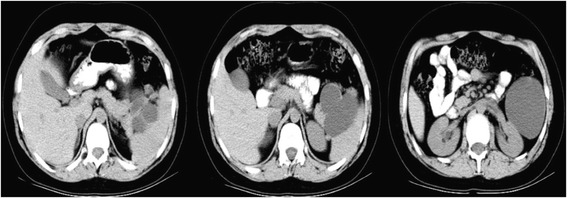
A computed tomography scan showing a cystic mass (10x7x9cm) with multiple septums located in the splenic hilum, tightly adjacent to the pancreatic tail and the left kidney.
